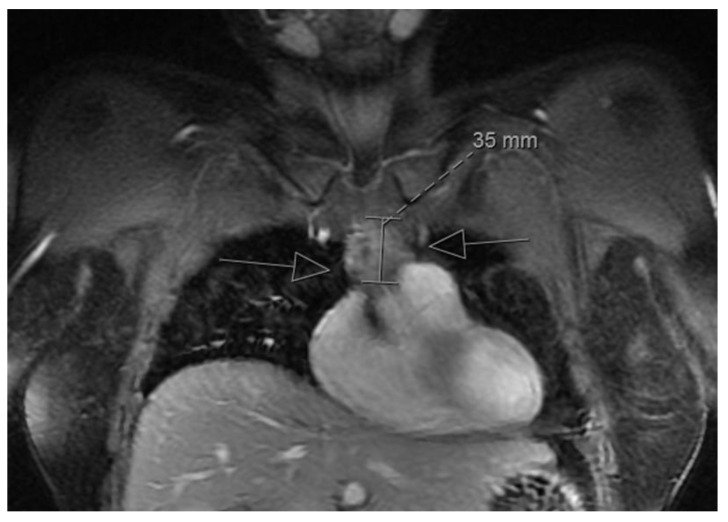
Magnetic resonance imaging in a LESA-like TH patient: Mediastinal mass-forming lesion with a diameter of 35 mm (arrows) in a patient with LESA-like TH (incidental finding).
